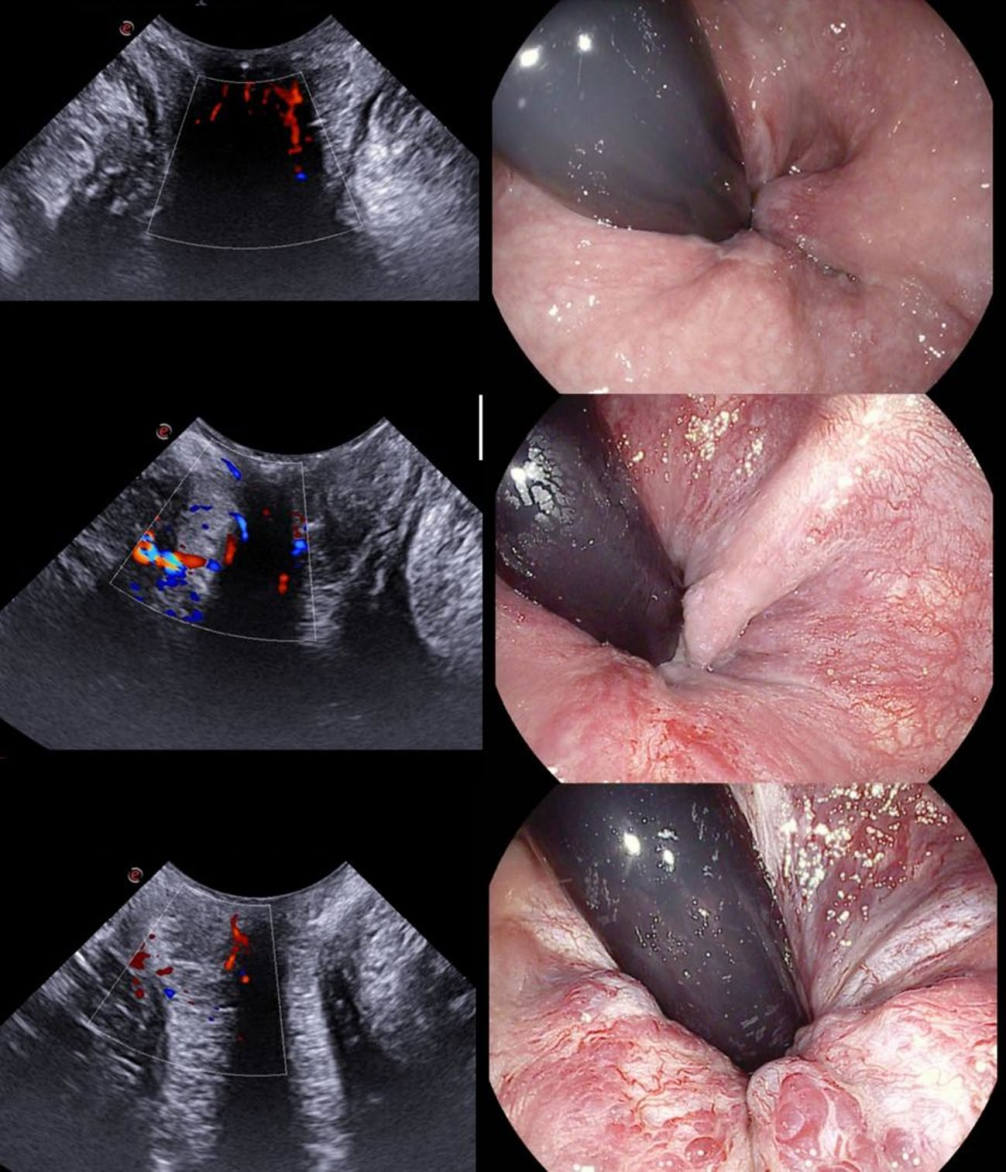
Comparison of the vascular pattern on transperineal US Doppler (*left panels*) with corresponding endoscopic images (*right panels*)
